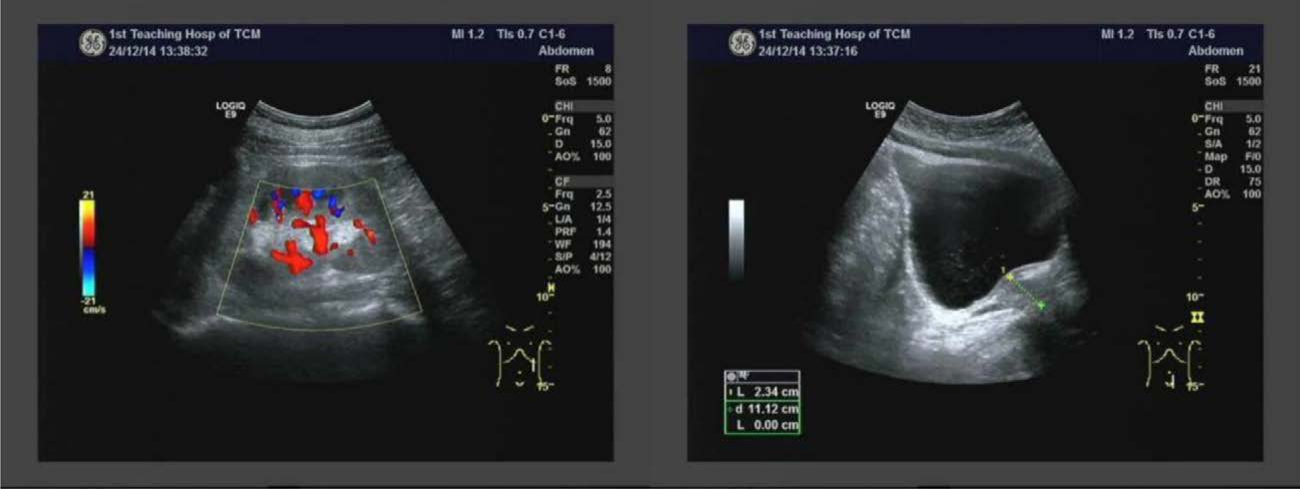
Urinary color doppler image.
